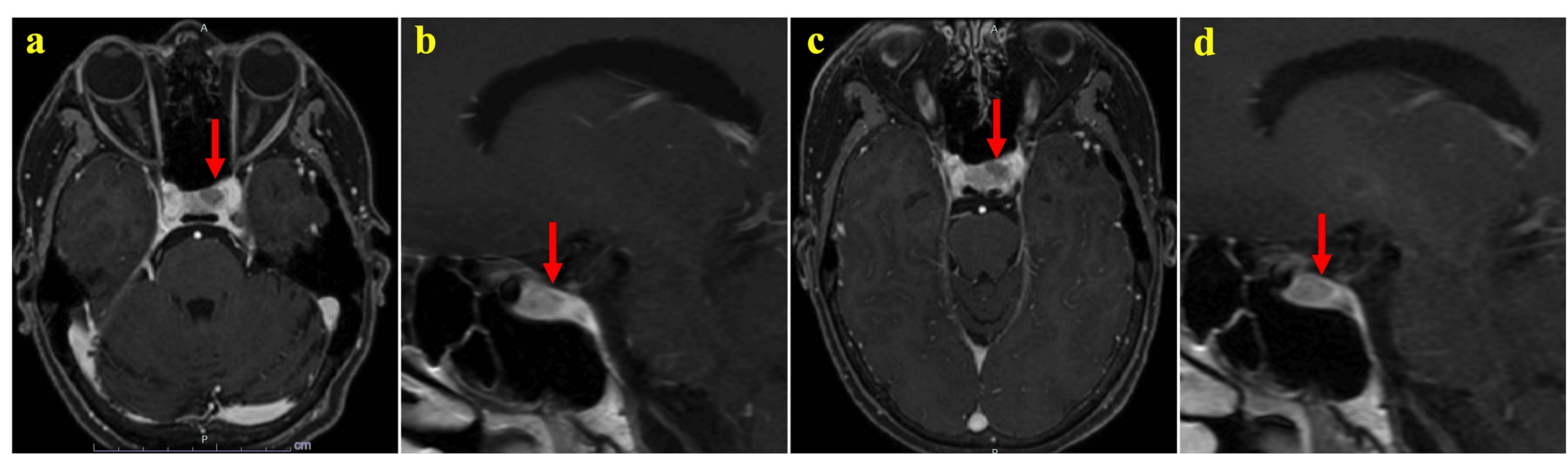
Pituitary magnetic resonance imaging Contrast-enhanced images are shown. a and b: Axial and sagittal views, respectively, taken on admission, show a 6 mm-diameter nodule with poor contrast effect (arrows). c and d: axial and sagittal views, respectively, taken seven months after the initiation of lanreotide treatment and are similar in size to a and b (arrows).
